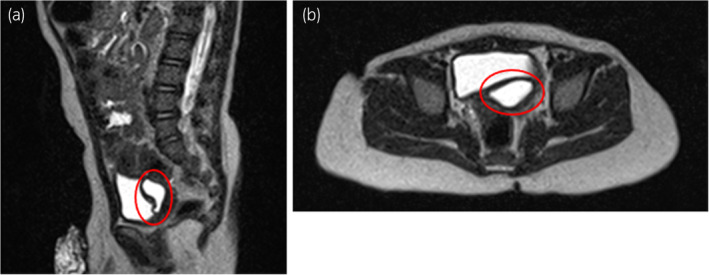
T2‐weighted MRI. (a) A 10‐mm low‐intensity lesion (red circles) is seen on the dorsal side of the bladder in sagittal section. (b) A low‐intensity lesion is apparent on the left side in axial section.
